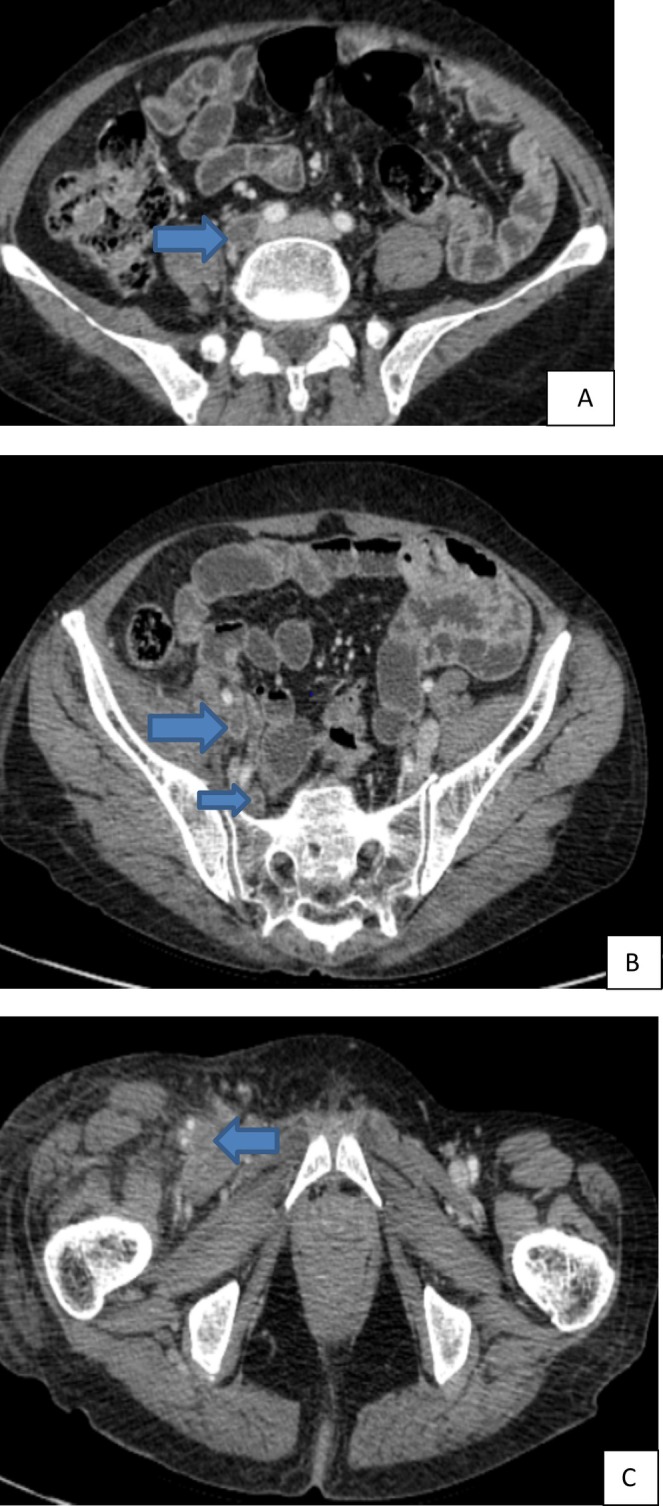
(A–C) Abdomino‐pelvic computed tomography showed: (A) a filling defect through the right common iliac vein; (B) internal (small arrow) and external (large arrow) iliac veins; and (C) right femoral vein.
